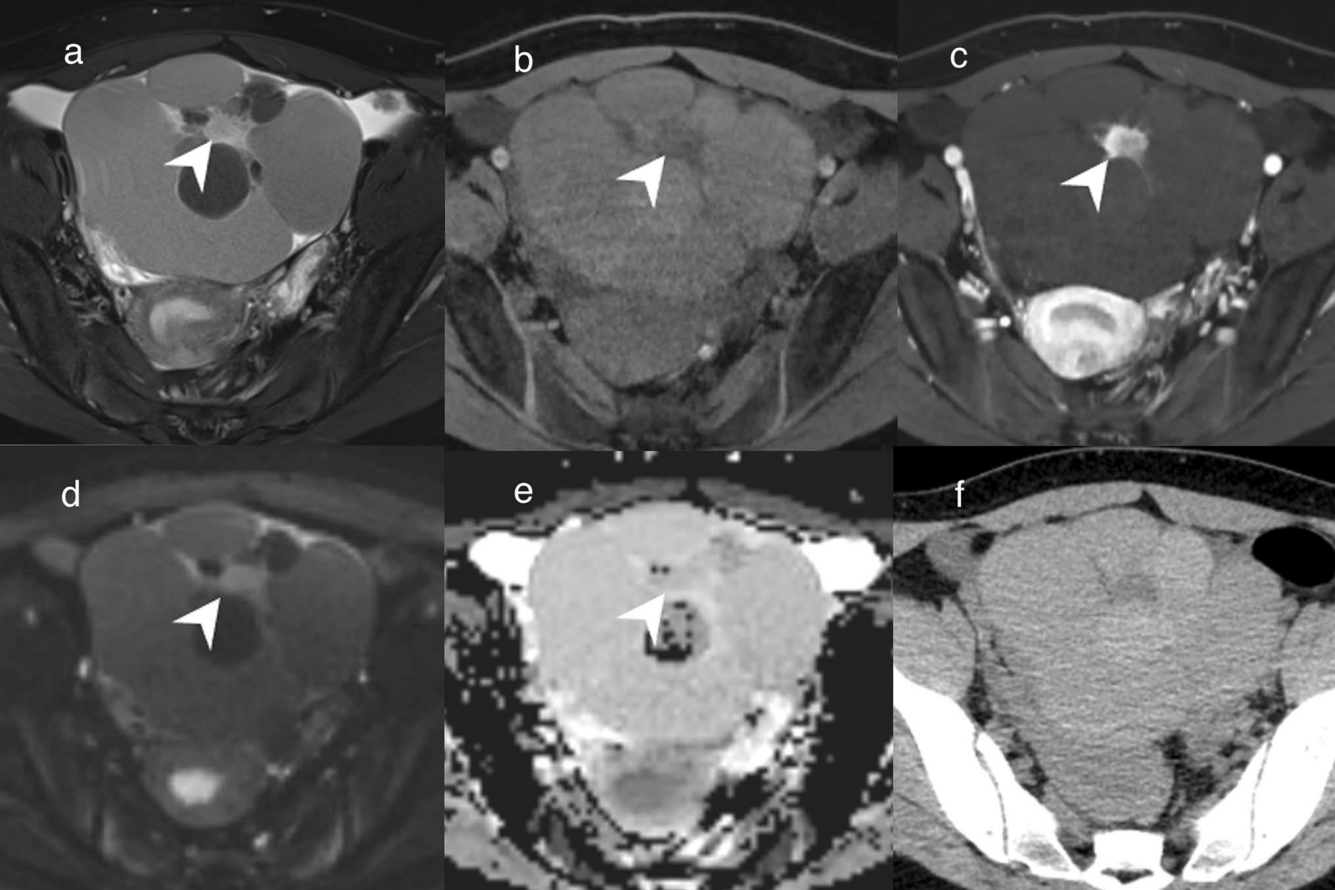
Struma ovarii in an adolescent girl. a FS-T2WI shows a lobulated multilocular cystic mass in the pelvis. Some loculi are as hypointense as skeletal muscle. A nodular area with hyperintensity is observable in the center of the mass (arrowhead). b On FS-T1WI, the majority of the mass is slightly hyperintense compared with skeletal muscle. A nodular area in the center of the mass is hypointense (arrowhead). c Gd-enhanced FS-T1WI shows no enhancement in the majority of the mass, but marked enhancement in the central nodular area (arrowhead). d The central nodular area is hyperintense on DWI (arrowhead) and ADC maps (e; arrowhead); hence, the area has no diffusion restriction. f Unenhanced CT shows the majority of the mass to be hyperdense compared with skeletal muscle, with the exception of the central nodular area
